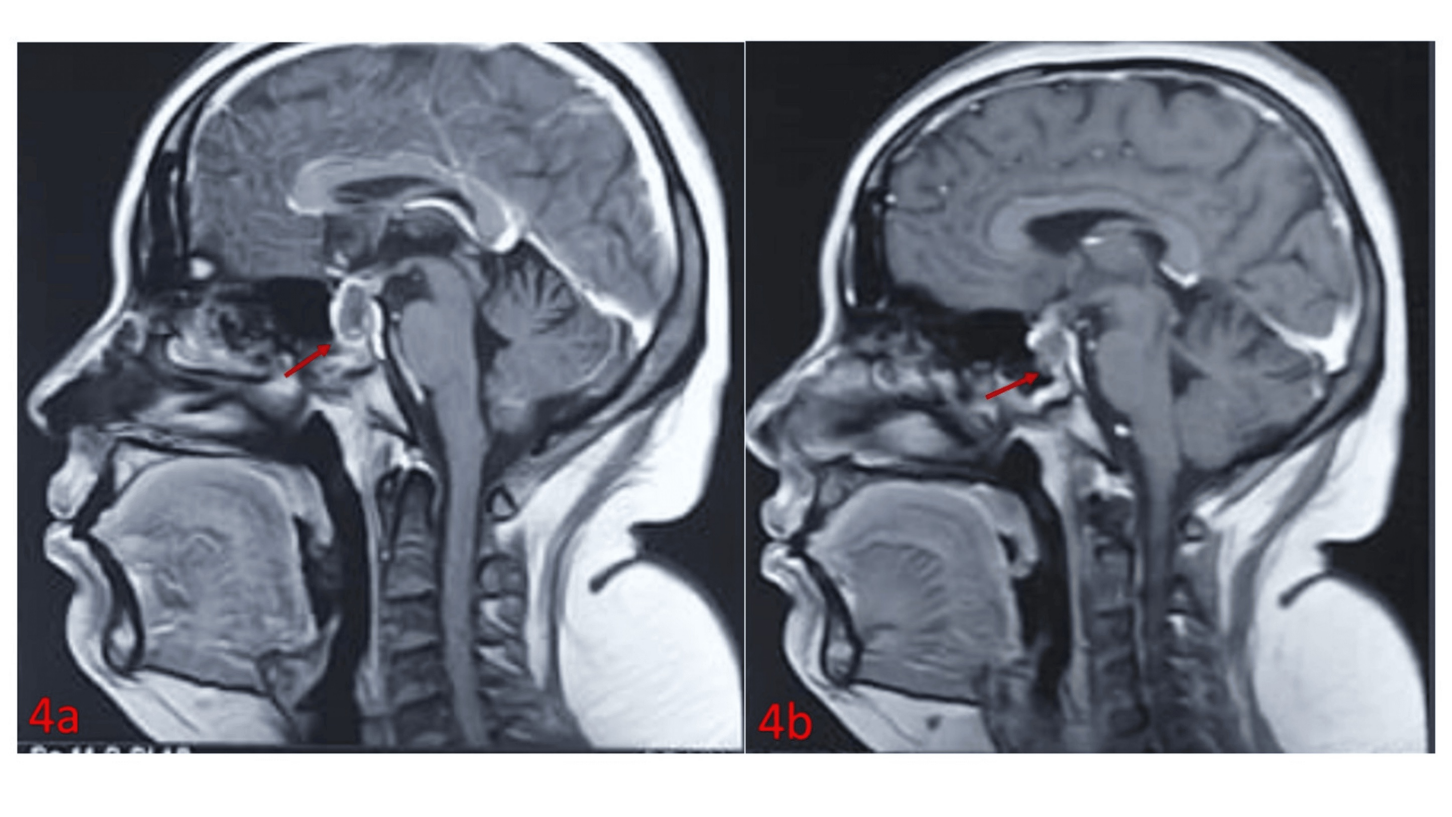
Sagittal T1-weighted contrast-enhanced MRI brain images (a–c) showing pituitary involvement in GPA The images reveal a homogeneously enhancing sellar mass with peripheral rim enhancement (red arrows), thickening of the pituitary stalk, and mild superior compression of the optic chiasm. GPA: granulomatosis with polyangiitis
